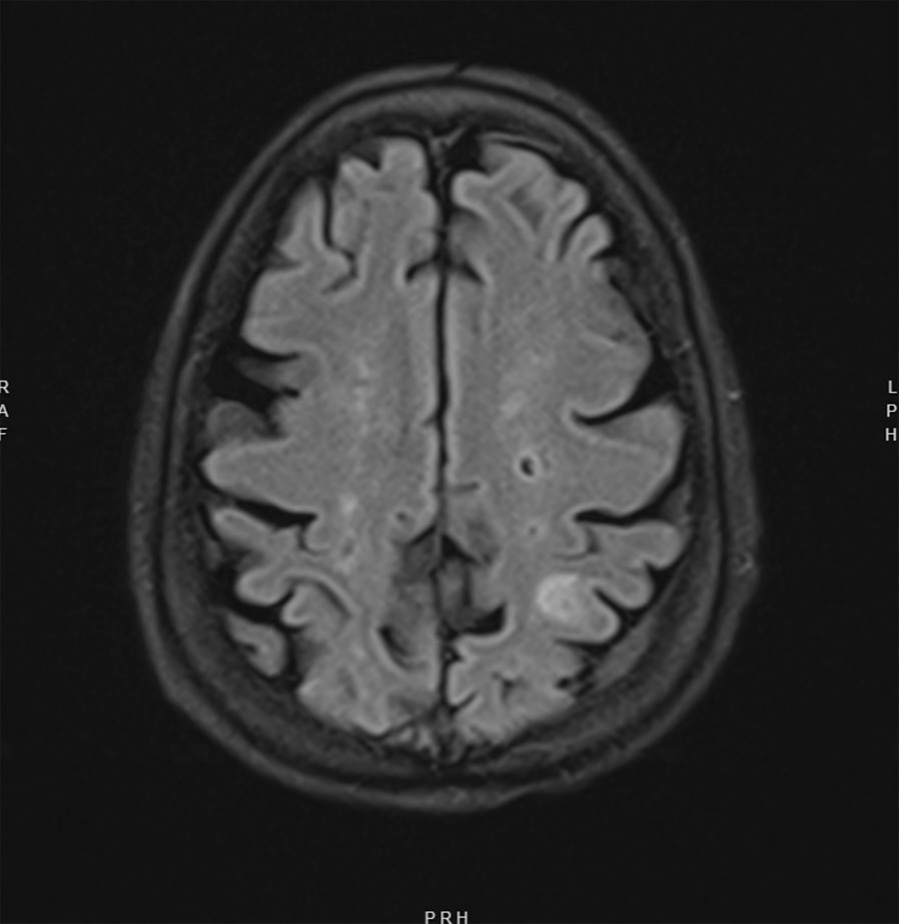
MRI Brain demonstrates prominent new left sided frontal and parietal foci consistent with small acute infarction and septic emboli
